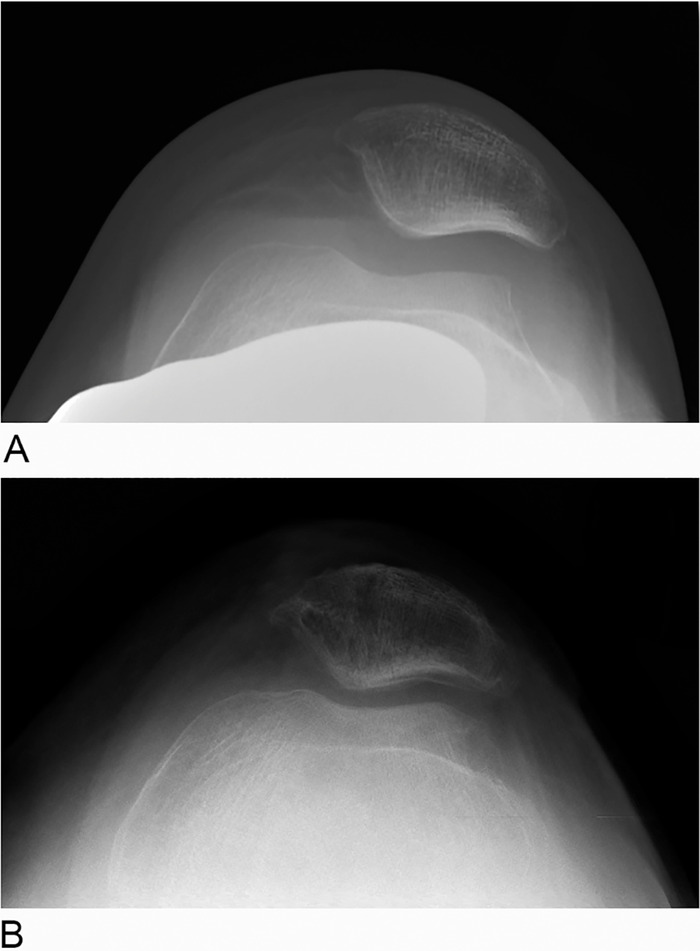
(A) Preoperative merchant view x-ray of the patient's left knee demonstrates maltracking. (B) Postoperative merchant view x-ray of the patient's left knee demonstrates improvement in articular constraint and tracking.
